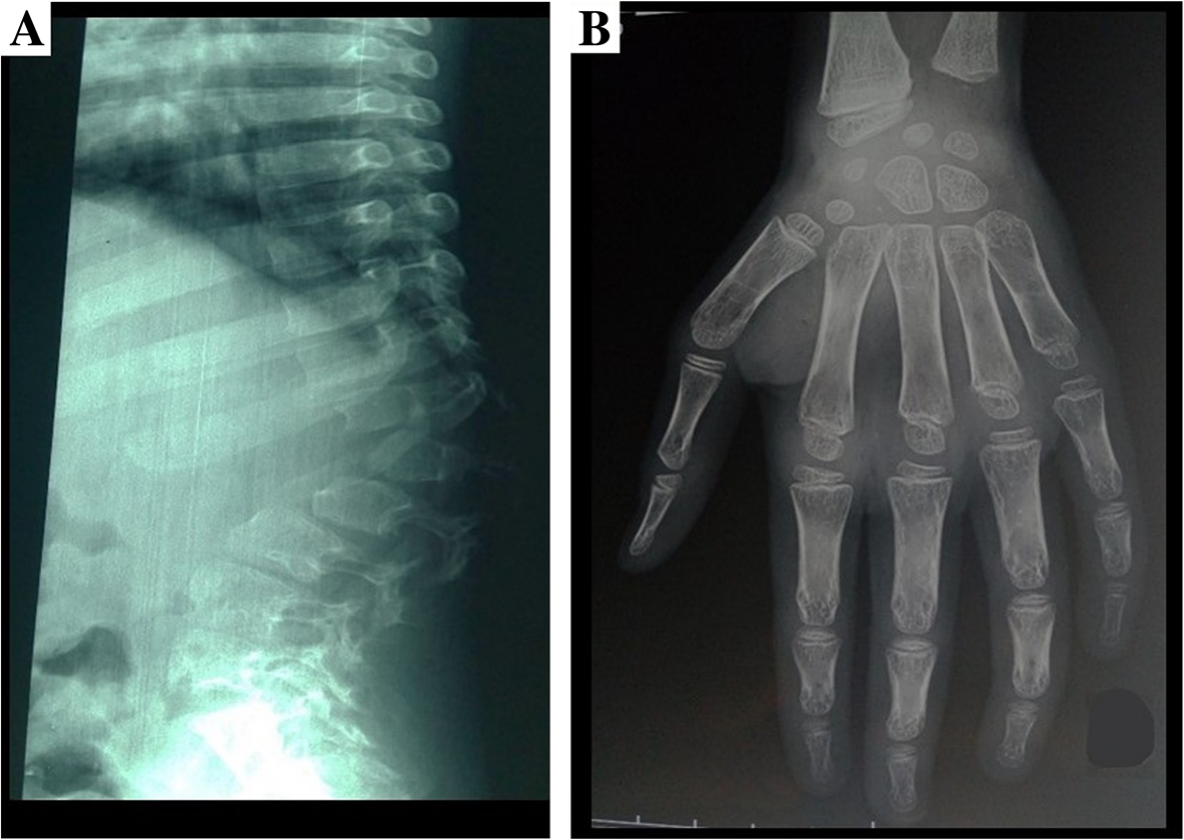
Skeletal X-rays showing anterosuperior hypoplasia of the vertebrae D12, L1, and L2 with kyphosis (a), and conical aspect of the distal ends of the phalanges (b)
